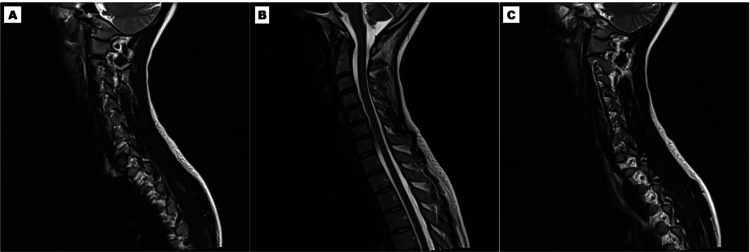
MRI Findings of Right C5-C6 Disc Herniation (Case 2) (A-C) Cervical MRI shows a right C5-C6 disc herniation without cord compression, offering only a partial explanation for the observed pain pattern.
